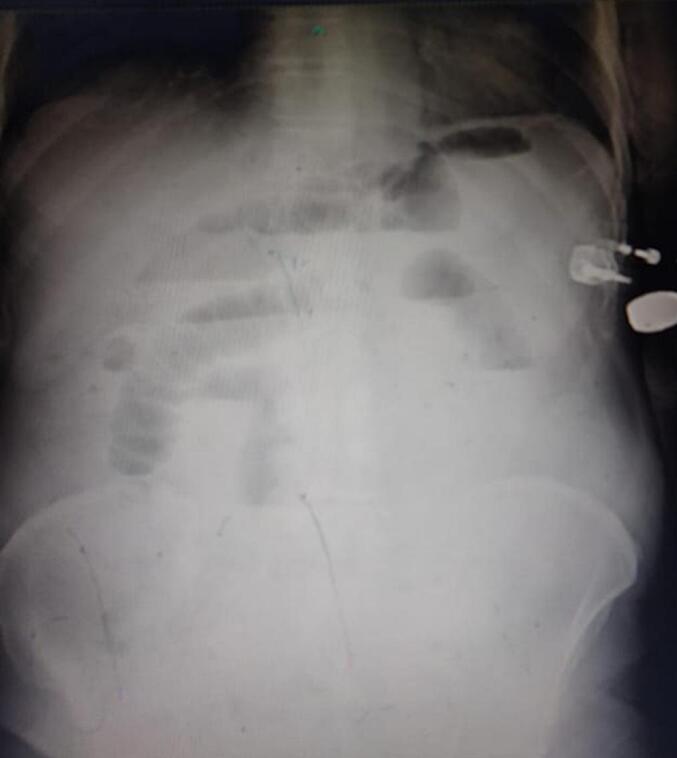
Erect abdominal x-ray showing multiple Air-Fluid levels.
